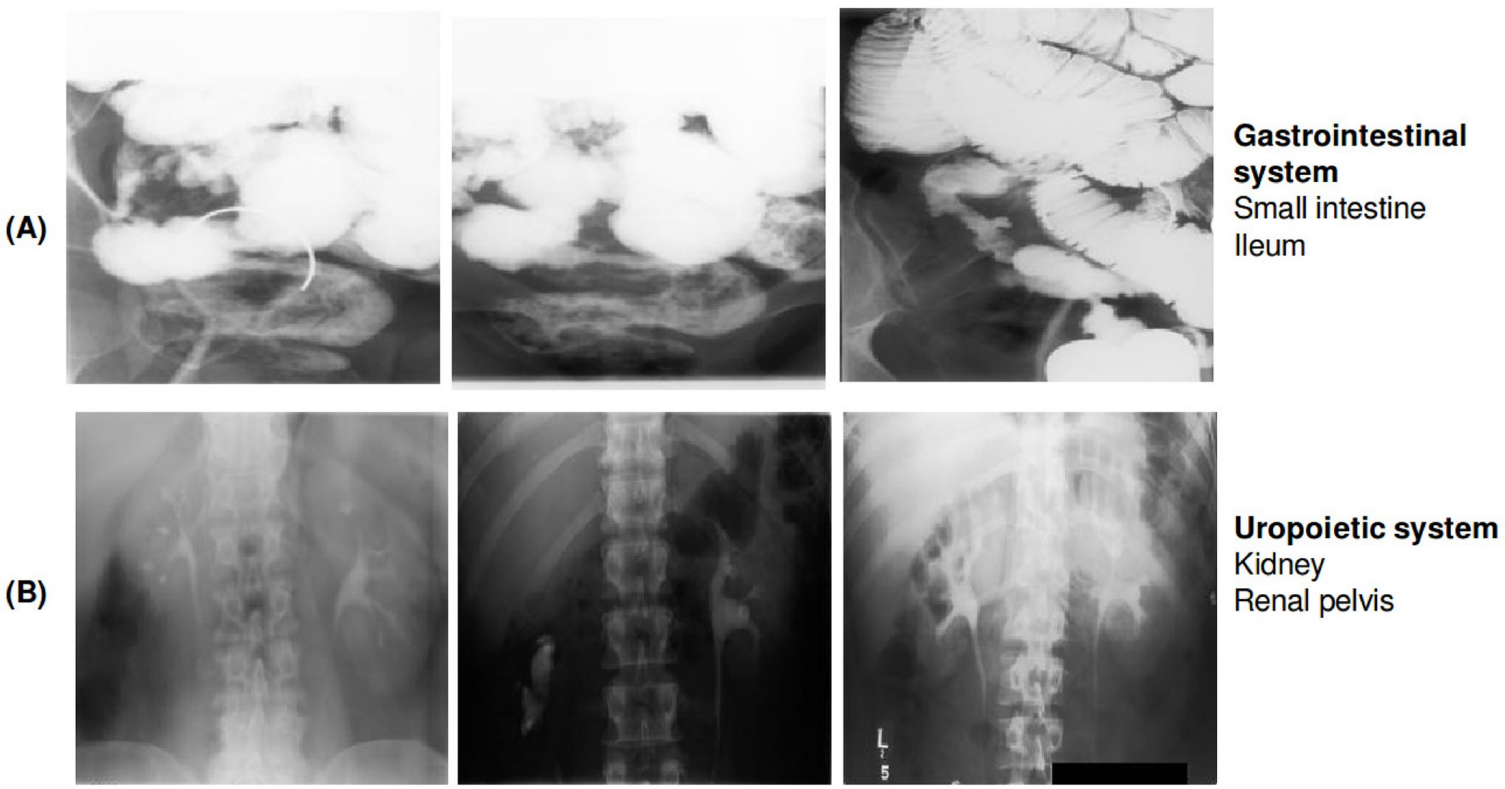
Examples of radiographs annotated with two classes from the B-scheme. (A) shows three imagse belonging to class ‘443’ representing ‘Gastrointestinal system; Small intestine; Ileum’ and (B) displays three images belonging to class ‘512’ representing ‘Uropoietic system; Kidney; Renal pelvis’. All radiographs were randomly chosen from the ImageCLEF 2009 Medical Annotation Task Training Set. Republished from [21] under a CC BY license, with permission from [RWTH Aachen], original copyright [2009].
